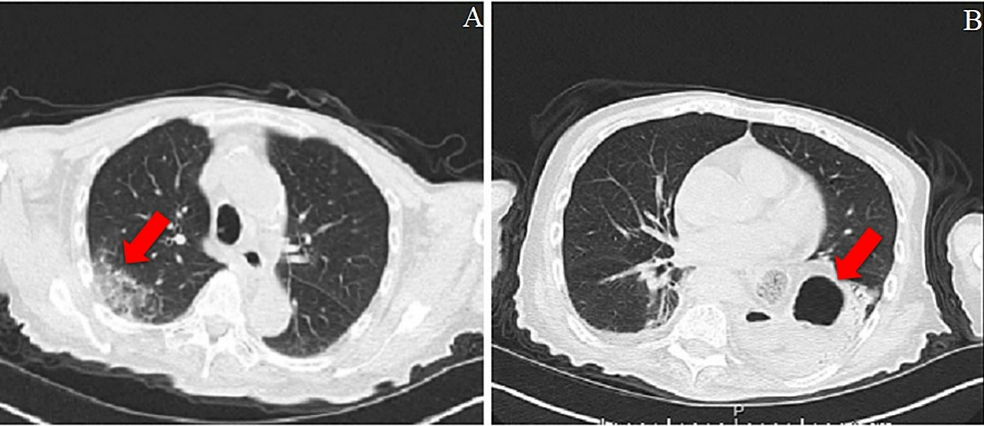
Chest CT scan of a 93-year-old male patient who died five days after his CT scan. A. COVID-19 pneumonia. B. Left-sided hiatal herniation.
